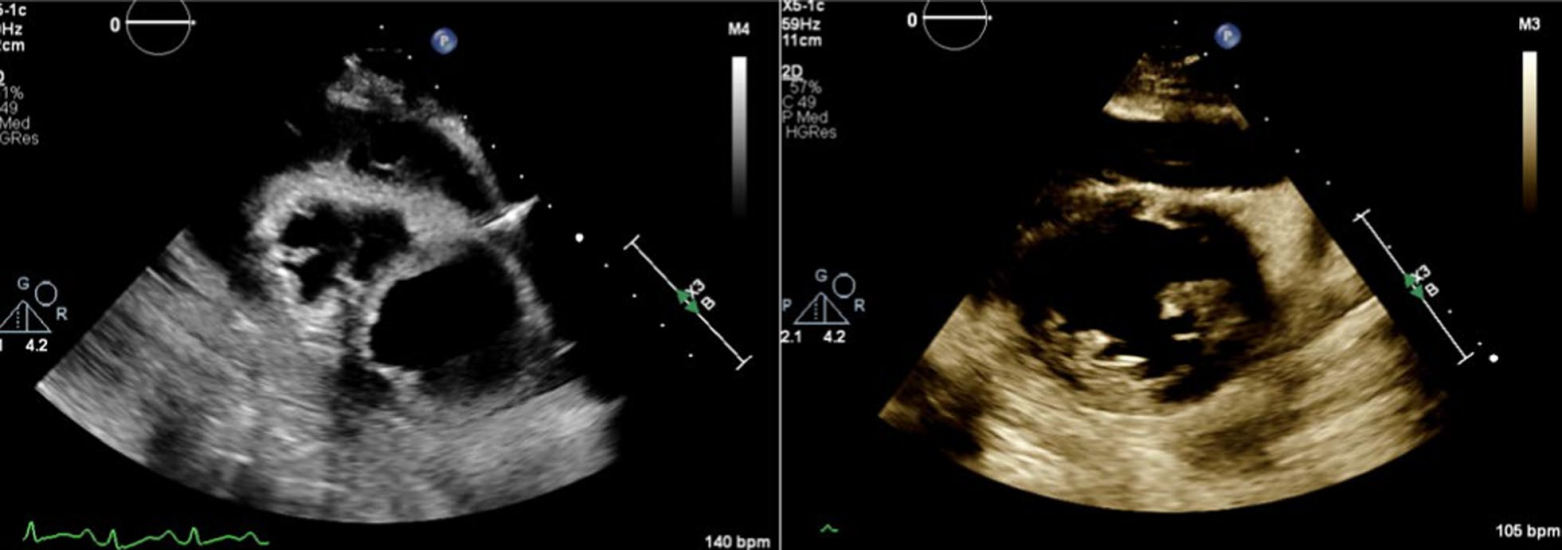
Case #1; TTE parasternal short-axis view before (left image) and after (right image) pericardiocentesis
